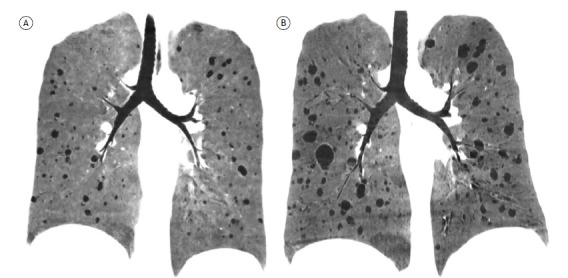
Evolution of the cystic pulmonary manifestation of lymphangioleiomyomatosis. Coronal CT scans of the chest, with minimum-intensity projection reconstruction, obtained at baseline (A) and at five years after diagnosis (B), showing progression of the condition, with increases in the number and size of the cysts.
